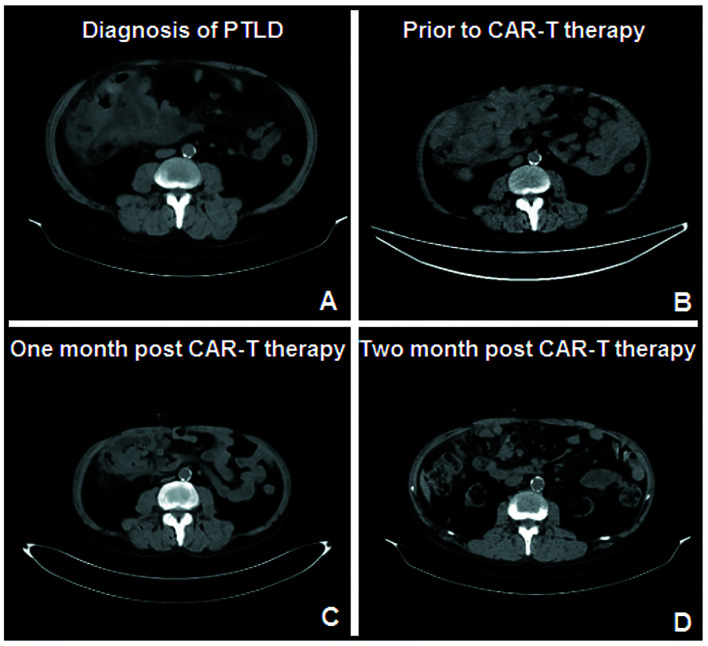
Results of the abdominal CT throughout the course of treatment. (A) Large area of mixed density in the right middle and lower abdomen when the patient was diagnosed with PTLD. (B) Before the anti-CD19-CAR T cell combined with PD-1 inhibitor therapy, he was refractory to all the combined chemotherapies. (C, D). One and 2 months after the combination therapy, CT scan showed a significantly smaller abdominal mass.
